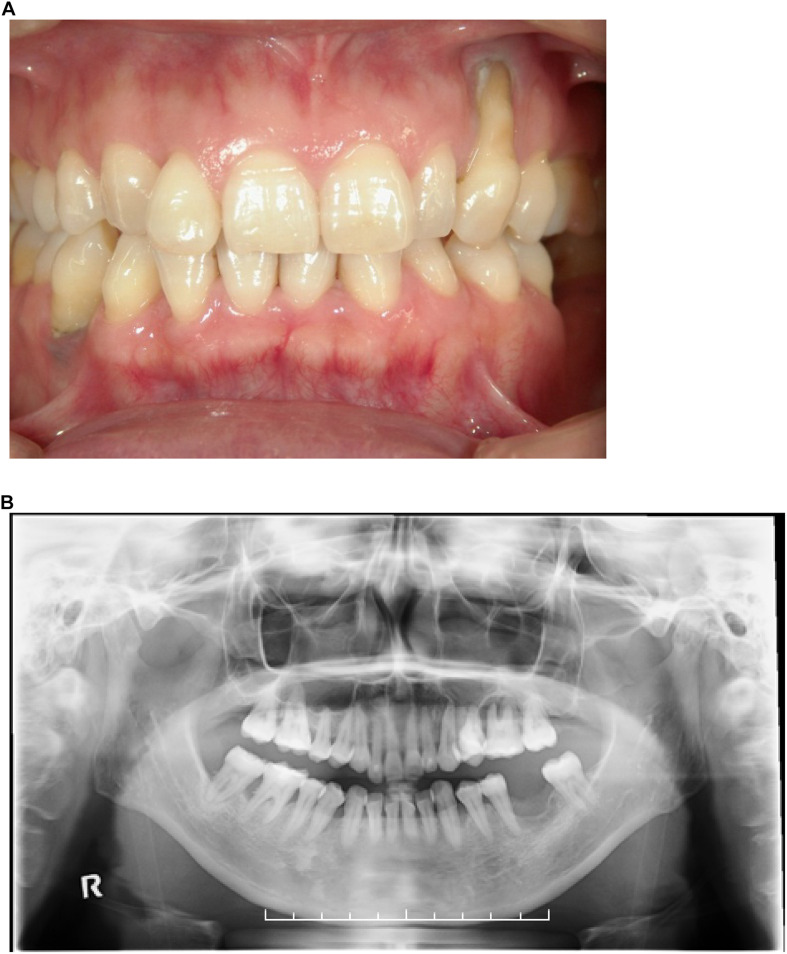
(A) Front view of the dentition of a patient with LDS and aggressive periodontitis. (B) Orthopantomograph of the patient. Note the presence of localized vertical bone loss around molars.
